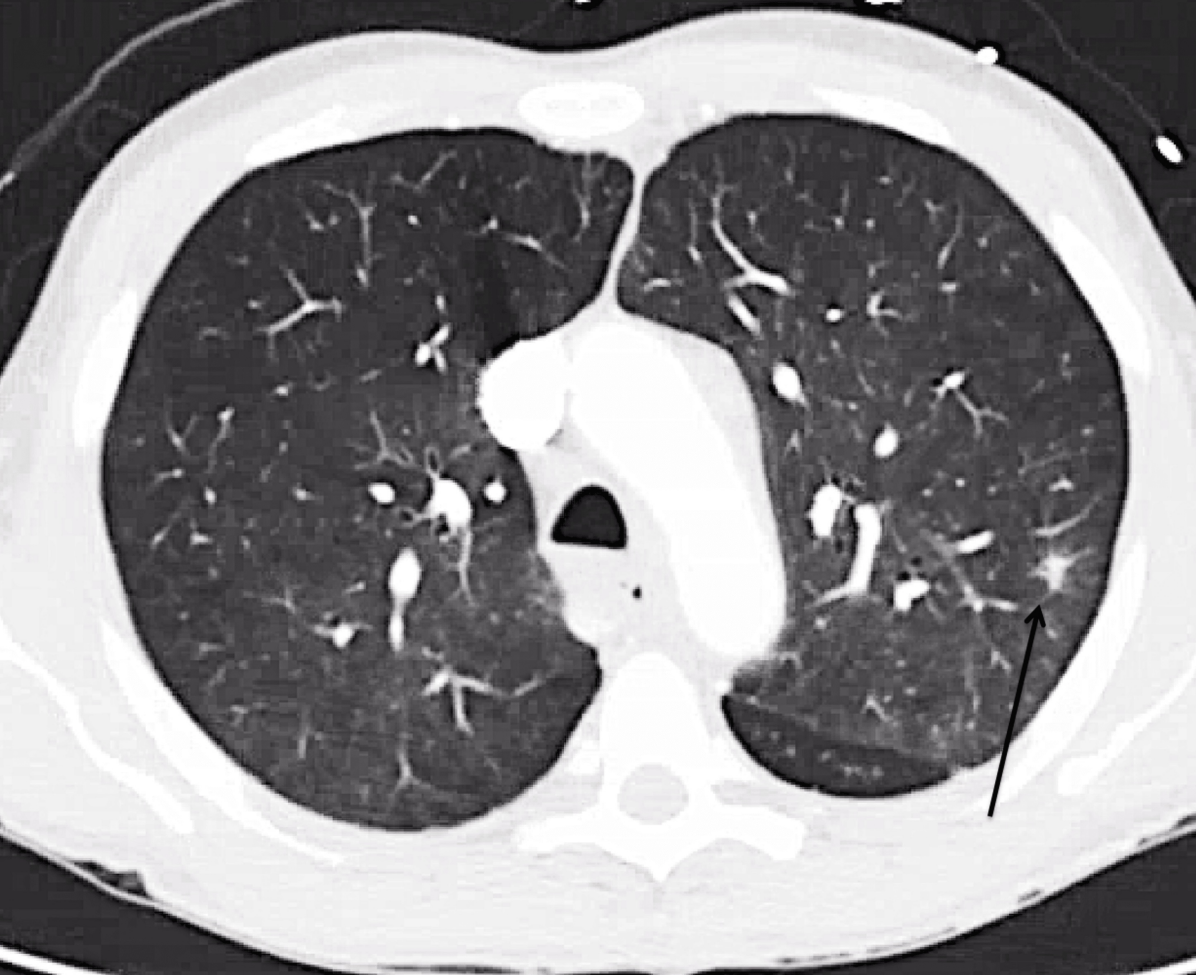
Computed tomography scan of the chest showing a spiculated lung nodule
